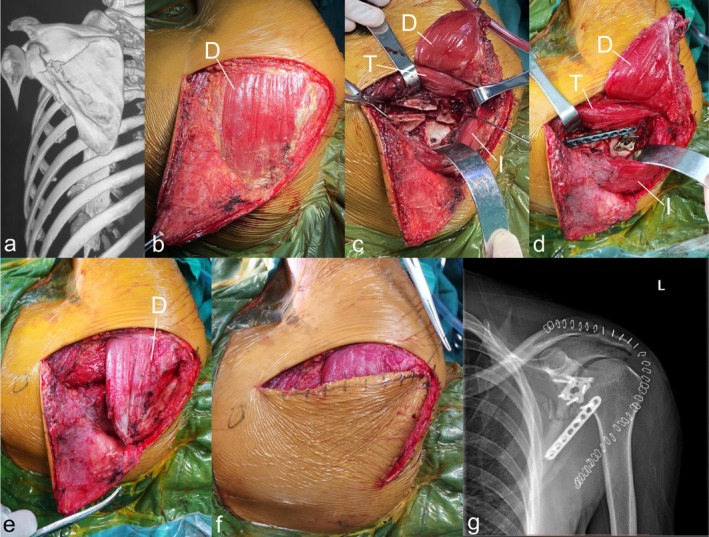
A 57‐year‐old male patient underwent treatment via the reverse Judet approach. (a) Preoperative 3D CT scan revealed a comminuted fracture of the scapular neck; (b–f) after muscle dissection, internal fixation and muscle flap suturing were performed (D: deltoid muscle, I: infraspinatus muscle, T: teres minor muscle); (g) postoperative X‐ray demonstrating restoration of normal anatomical structures.
